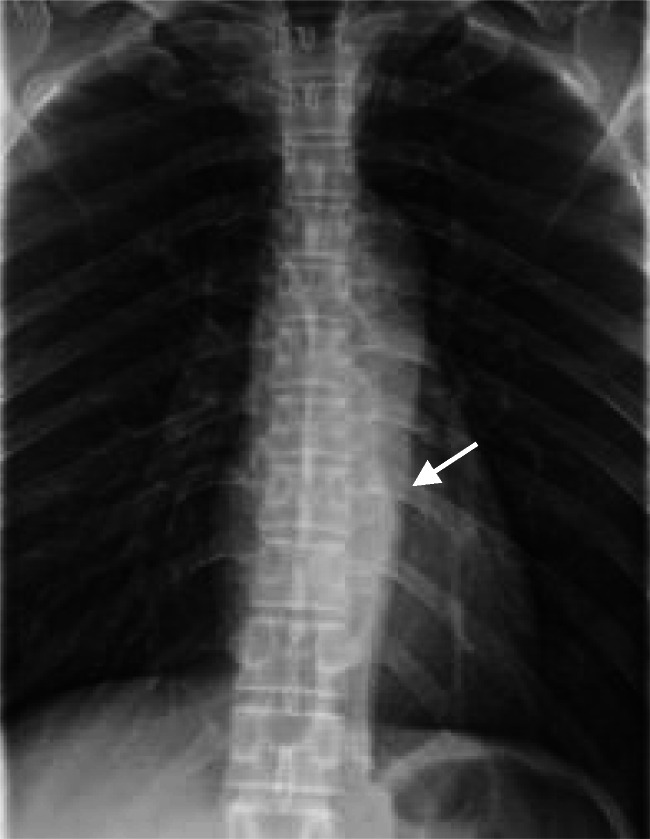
Antero–posterior view of the thoracic spine radiograph. Soft tissue density mass is visualized on the left of Th9–10 (*white arrow*). The mass makes the left transverse process and posterior rib indistinct. This suggests paravertebral lesion
